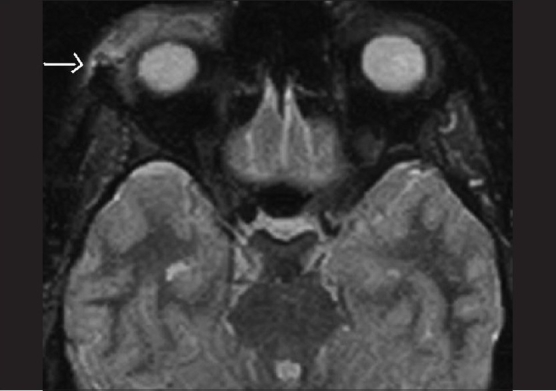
Repeat MRI fat suppressed STIR sequence showing increase in size of the lesion
